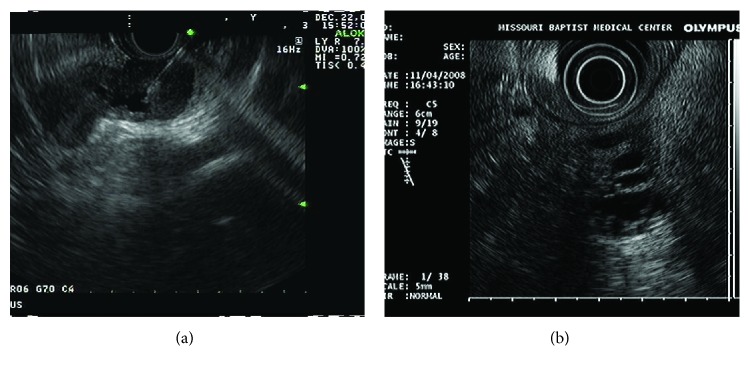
Endoscopic ultrasound imaging in a patient presenting with mucinous cystic lesion showing focal wall thickening.
